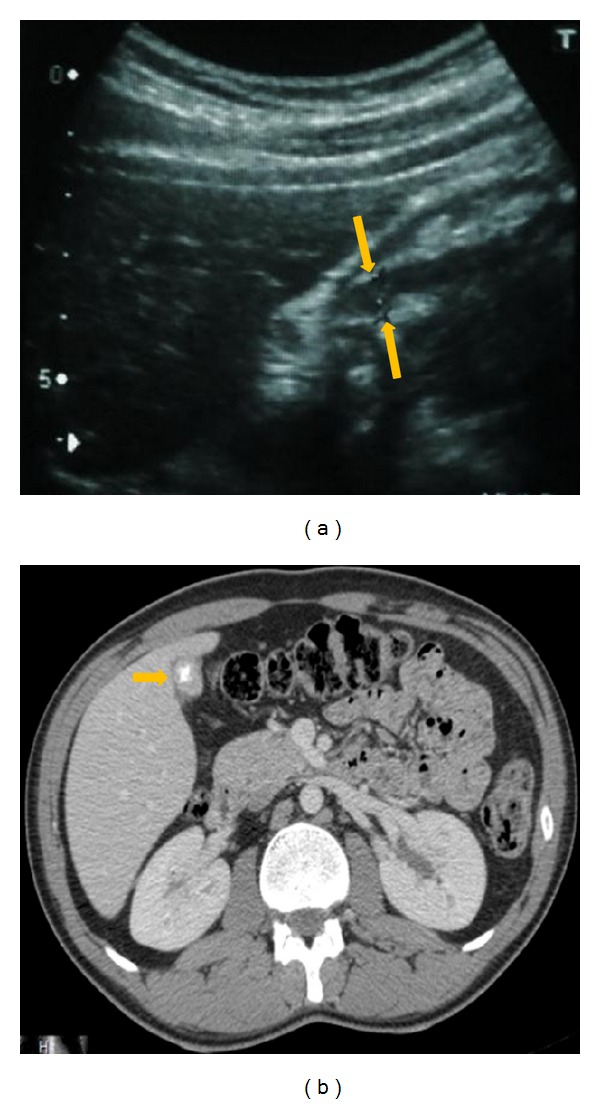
Ultrasound (a) and CT scan (b) showing the gallbladder was retracted with microlithiasis and diffuse and circumferential thickening of its wall.
